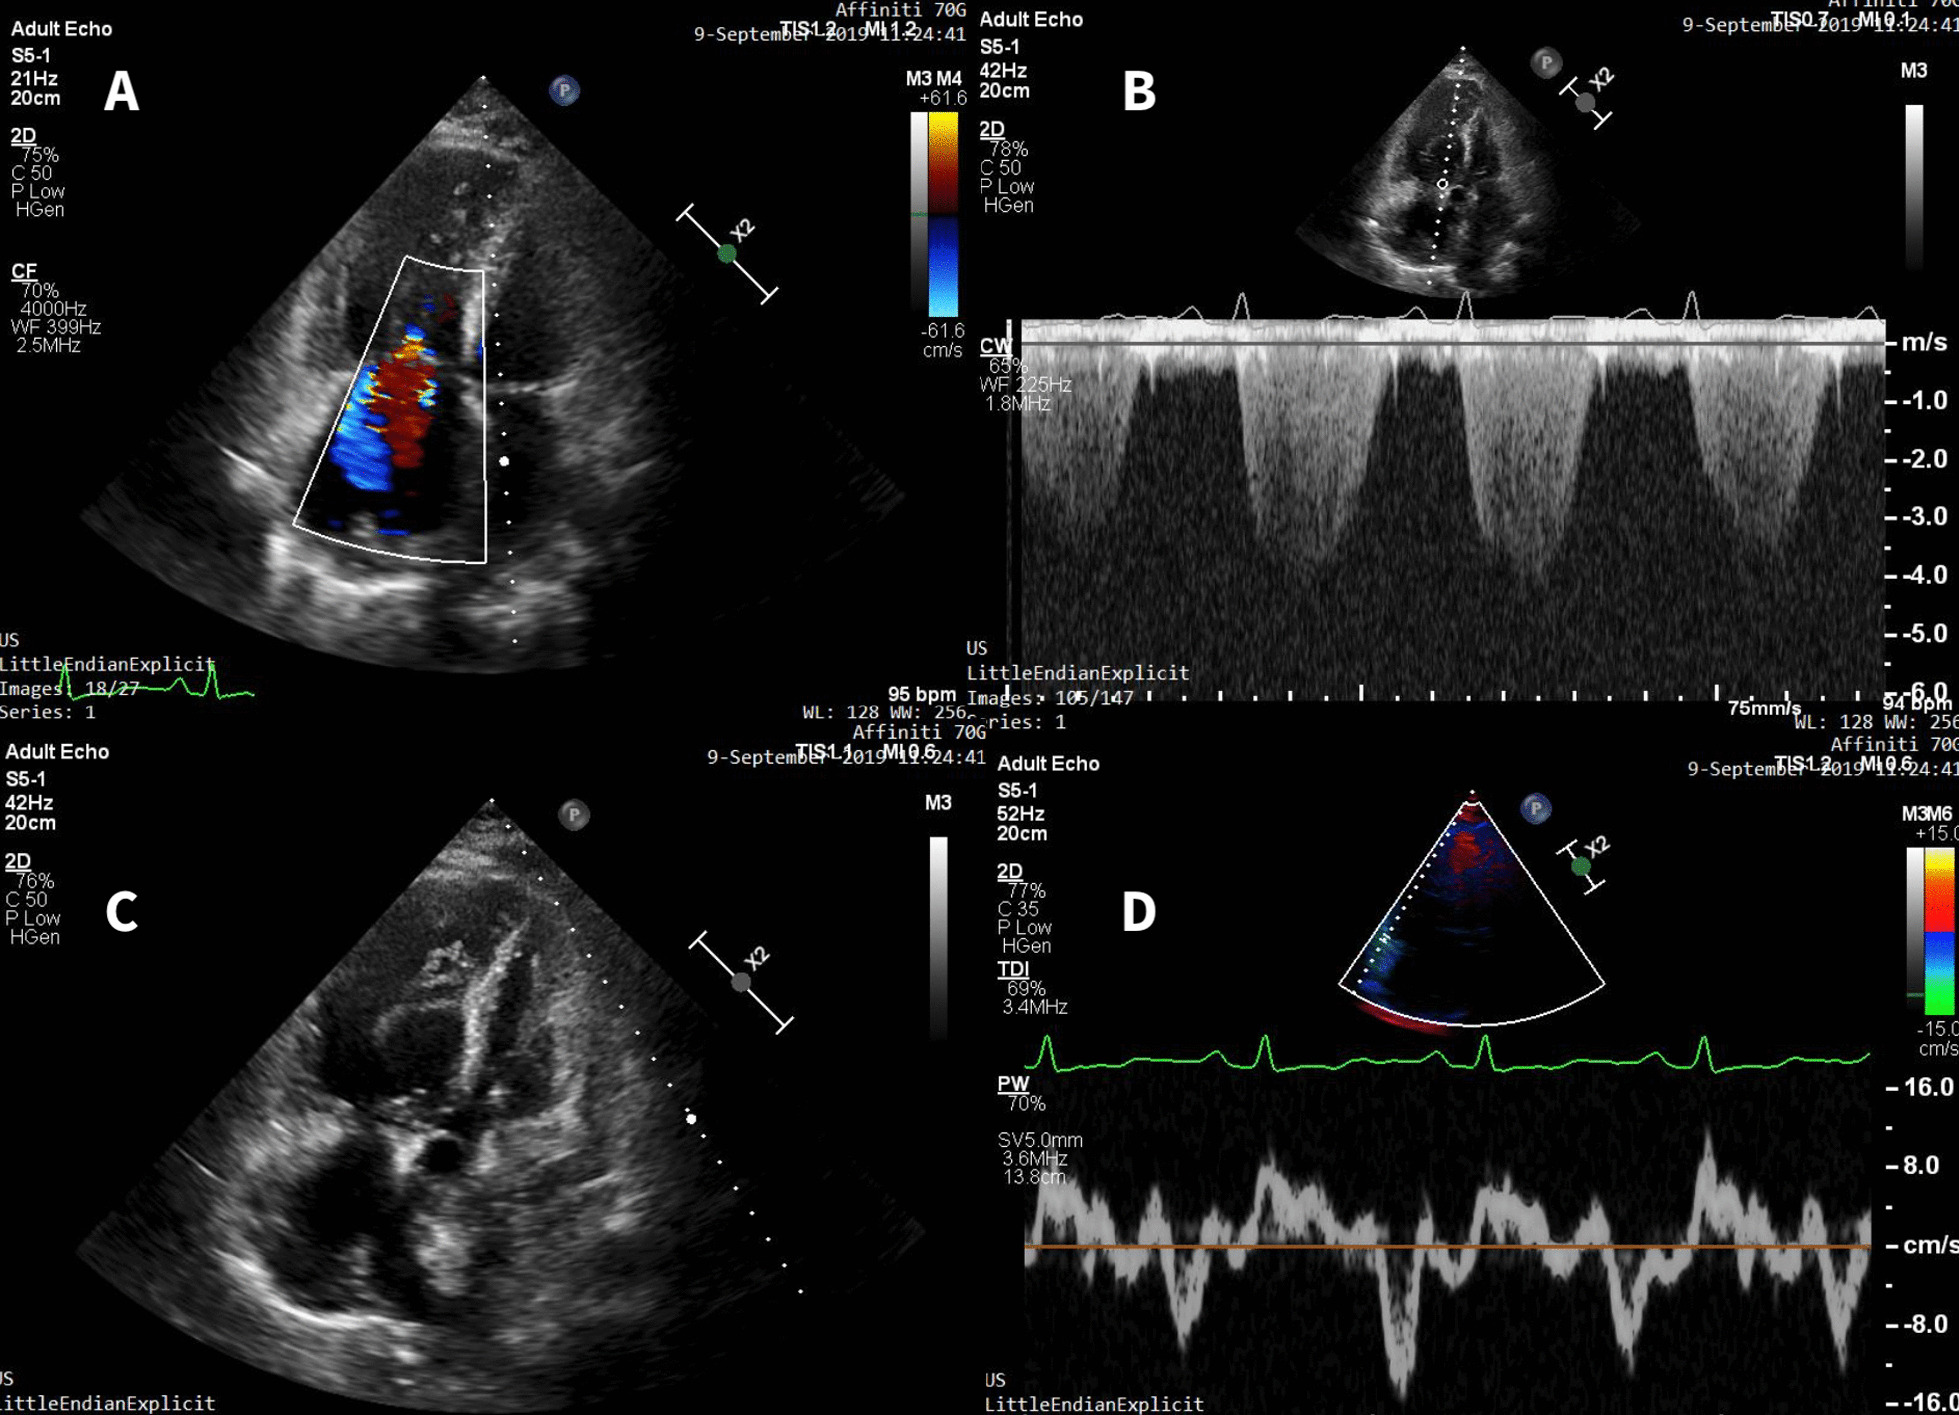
2D Doppler echocardiography—four chambers apical view A, B, C, D. Revealing tricuspid regurgitation, maximal tricuspid regurgitation velocity (TR Vmax)—4.1 m/s indicating pulmonary hypertension, right heart enlargement and reduced RV free wall longitudinal function (S’—8.0 cm/s)
